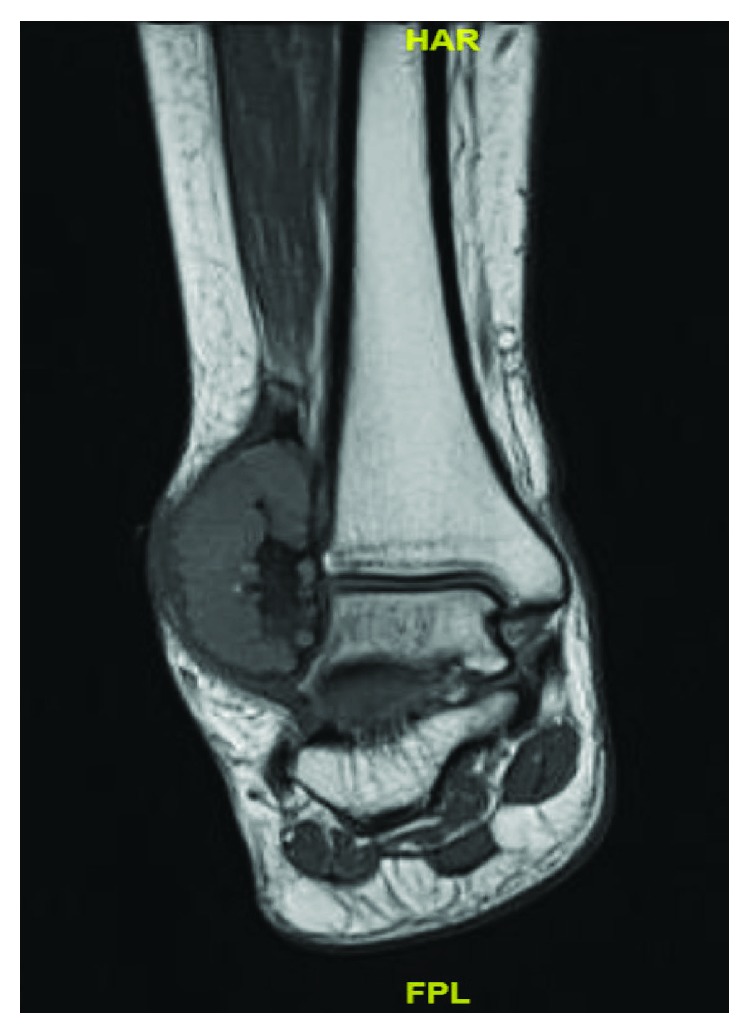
T1 weighted MRI showing a homogeneously slightly hyperintense to muscle lobulated lesion with multiple fluid-fluid levels.
